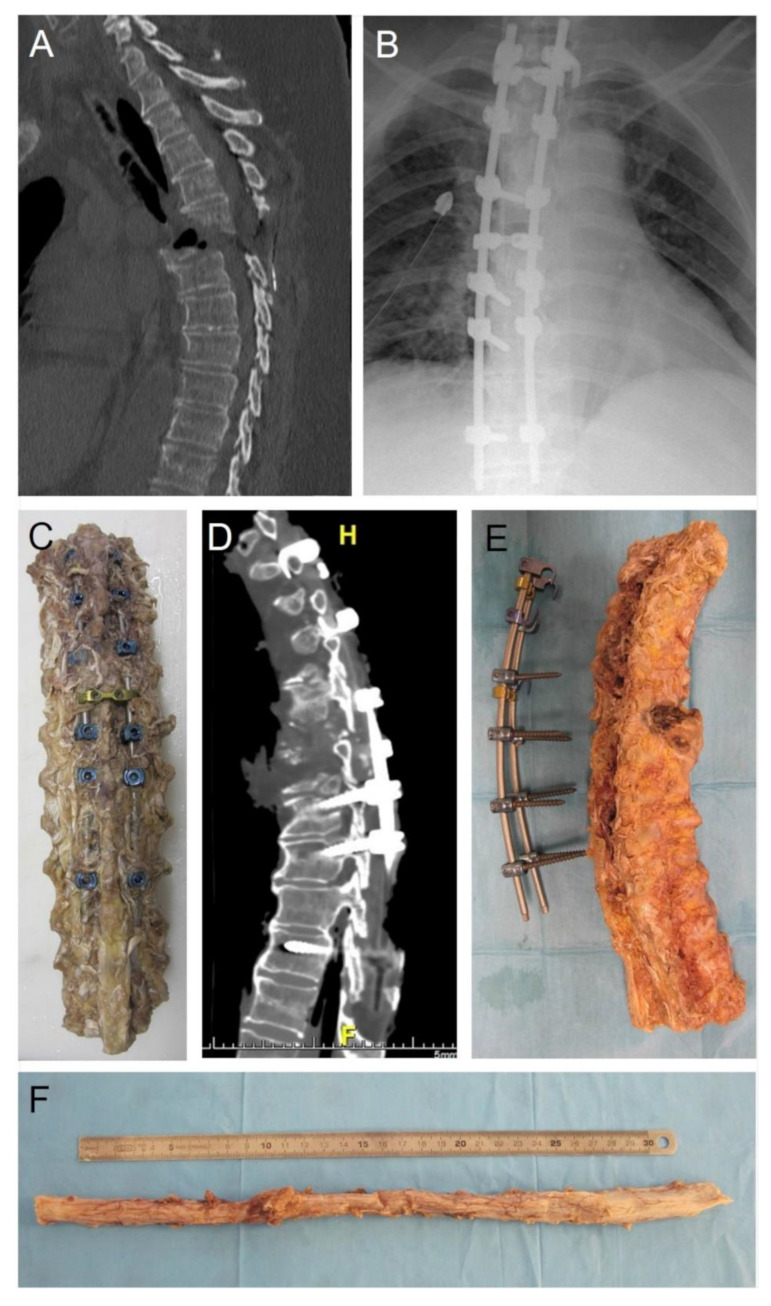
Spine trauma. (A) in vivo pre-surgery computed tomography. (B) in vivo post-surgery radiography. (C) sampling of the spine segment. (D) post-autopsy computed tomography of the sampled spine. (E) removing of pedicle screws. (F) general view of the spinal cord, with evidence of hemorrhagic infiltration of the dural sac. (H: Head; F: Feet).
